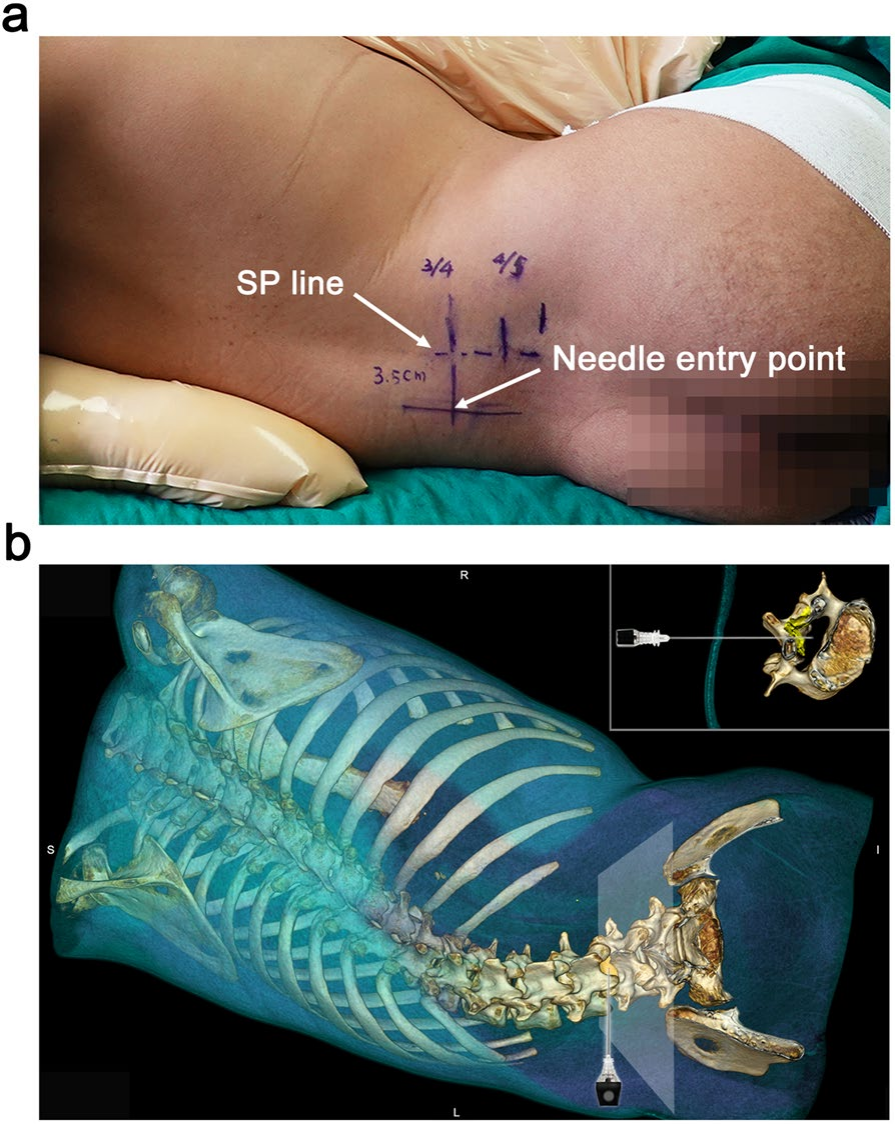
A horizontal needle path demonstrated by CT volume rendering reconstruction images. a The determined needle entry point (white arrow) of a SMA patient with scoliosis, 3.5 cm from the spinous process (SP) line. b Three-dimensional CT reconstruction in a volume rendering technique of this patient demonstrating the needle directed horizontally to the center of the dural sac on the convex side through the determined needle entry point at the L3 – L4 interspace. SMA, spinal muscular atrophy
